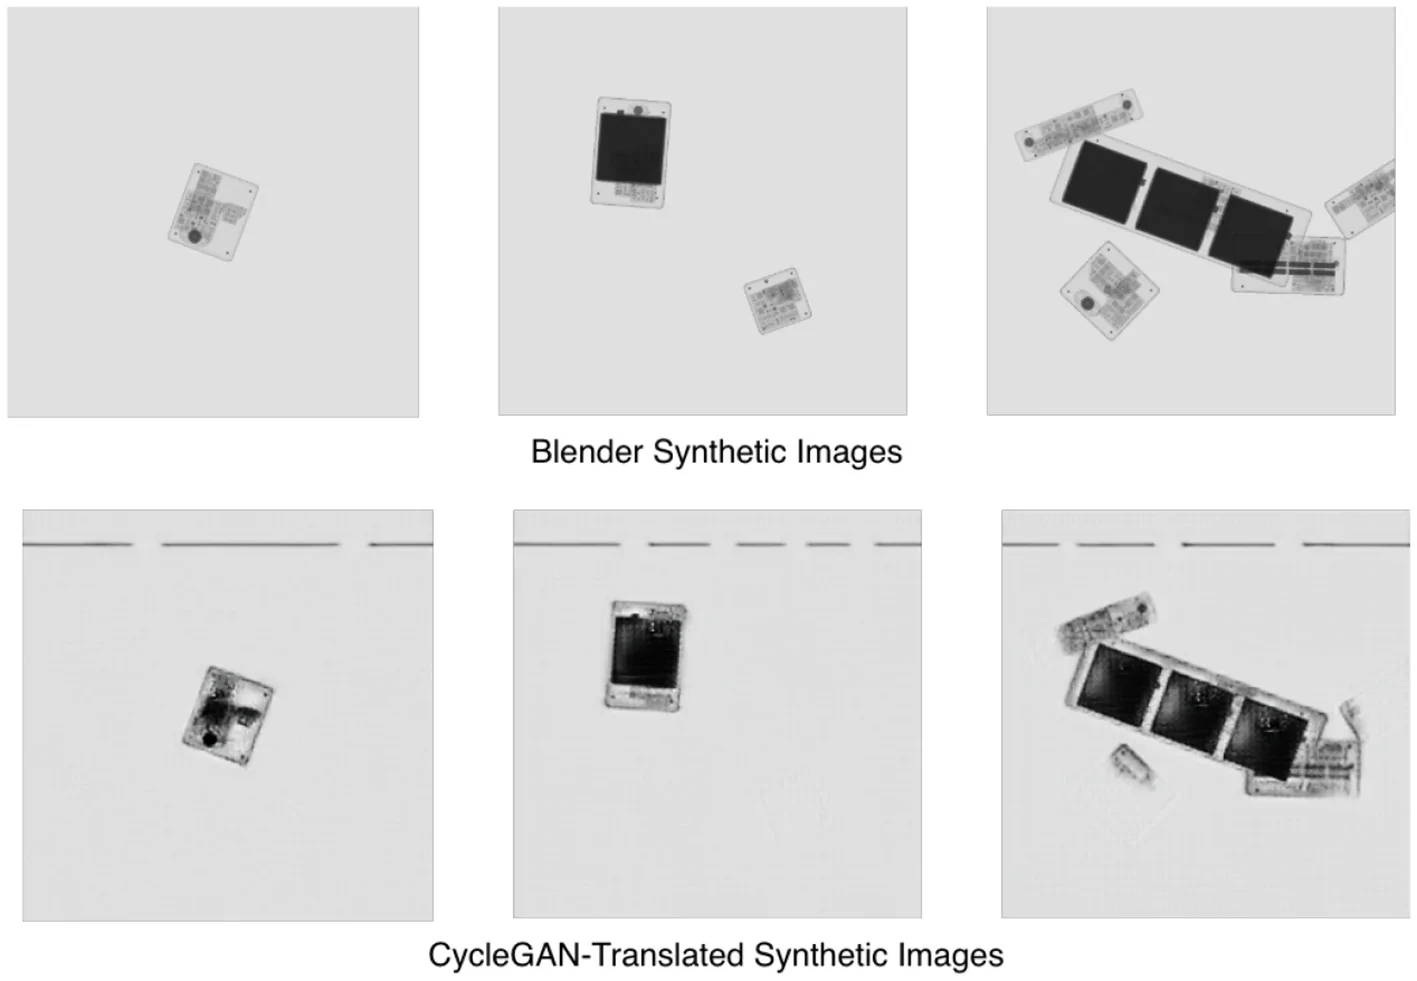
Qualitative comparison of Blender-rendered and CycleGAN-translated synthetic X-ray images.
